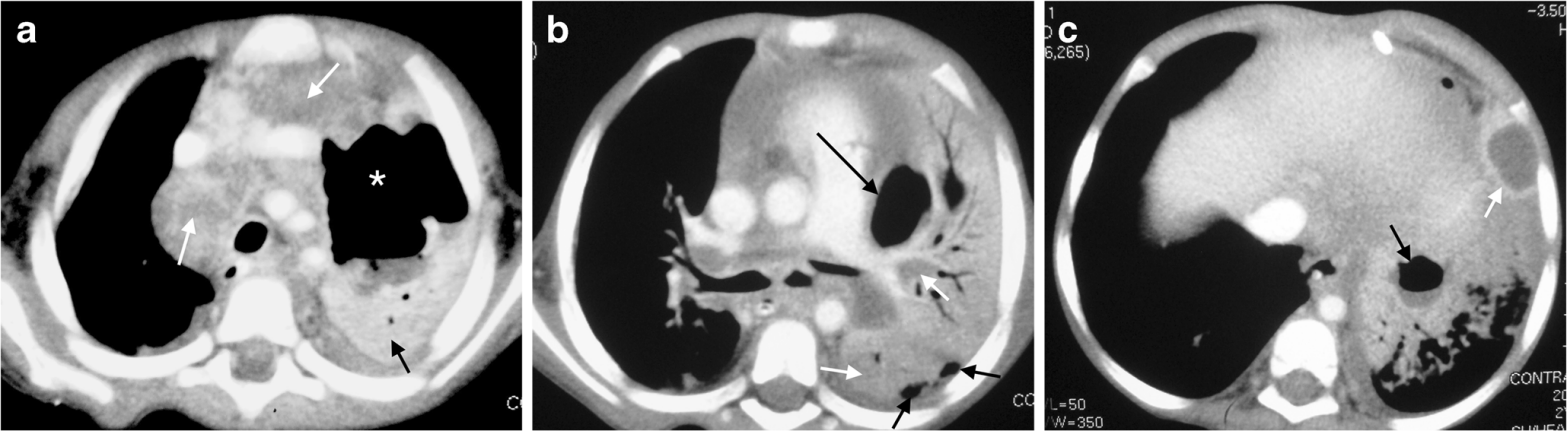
A 2-year-old girl with confirmed tuberculosis, demonstrating progression of lung necrosis. a Axial CT at the level of the main branches of the aorta demonstrates the typical low-density right paratracheal and anterior mediastinal lymphadenopathy (*white arrows*), associated with tuberculosis as well as a large air-filled cavity (*asterisk*) and fluid level in the left upper lobe due to lung necrosis. Some consolidated vital lung is seen enhancing posteriorly (*black arrow*). There is also bilateral effusion. b Axial CT at the level of the main pulmonary outflow tract demonstrates medial and posterior low density non-enhancing necrotic areas of the left consolidated lung (*white arrows*) as well as a medial cavity very closely associated with the pleural space (*long black arrow*). There are also small pockets of air in the pleural space posterior (*short black arrows*). c Axial CT at the level anterior diaphragm demonstrates cavitation of the left lower lobe with an air-fluid level (*black arrow*) as well as formation of an abscess seen as a clearly enhancing wall, surrounding a fluid collection (*white arrow*)
